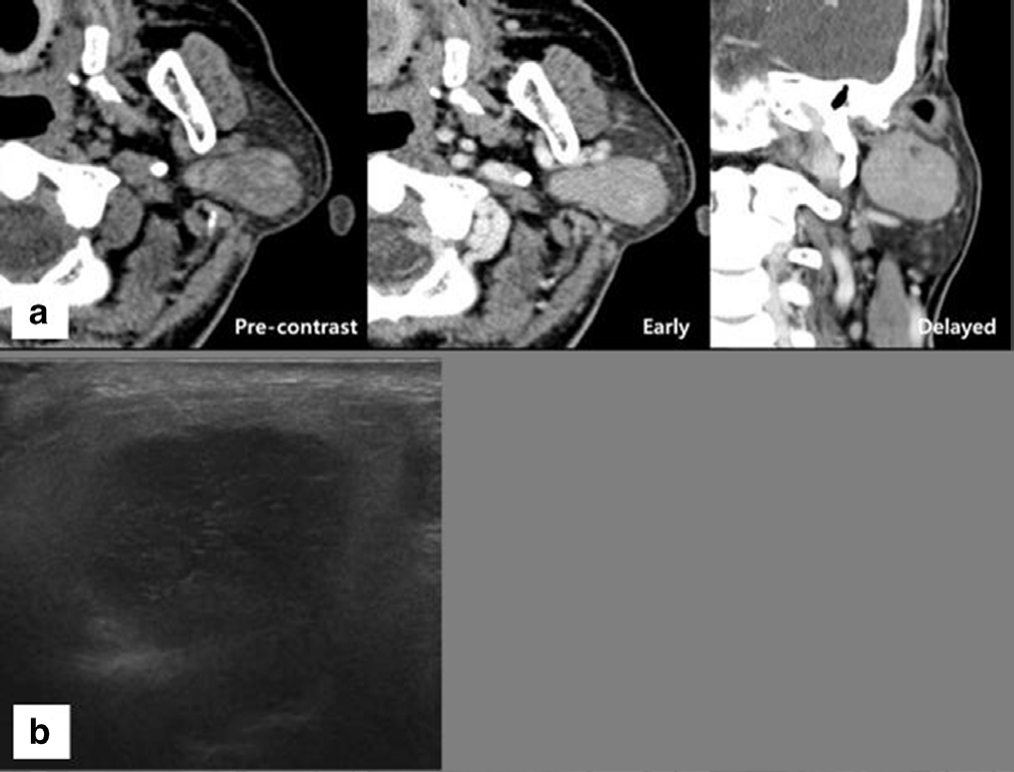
A 68-year-old male with slow-growing mass in the left preauricular area. (a) A well-marginated heterogeneously attenuated (32~78 HU) soft tissue mass measuring about 3 cm shows dumbbell shape involving both superficial and deep lobes of the left parotid gland on axial precontrast CT scan. After 60 s from the injection of contrast media, the tumor shows dense enhancement (100 HU) on axial CT scan. After 120 s from the injection of contrast media, no definite contrast-washout nor gradual enhancement of the mass (100 HU) is noted on coronal CT scan. (b) Sonogram shows a well-defined heterogeneous hypoechoic mass in the left parotid gland. HU, Hounsfiled unit.
